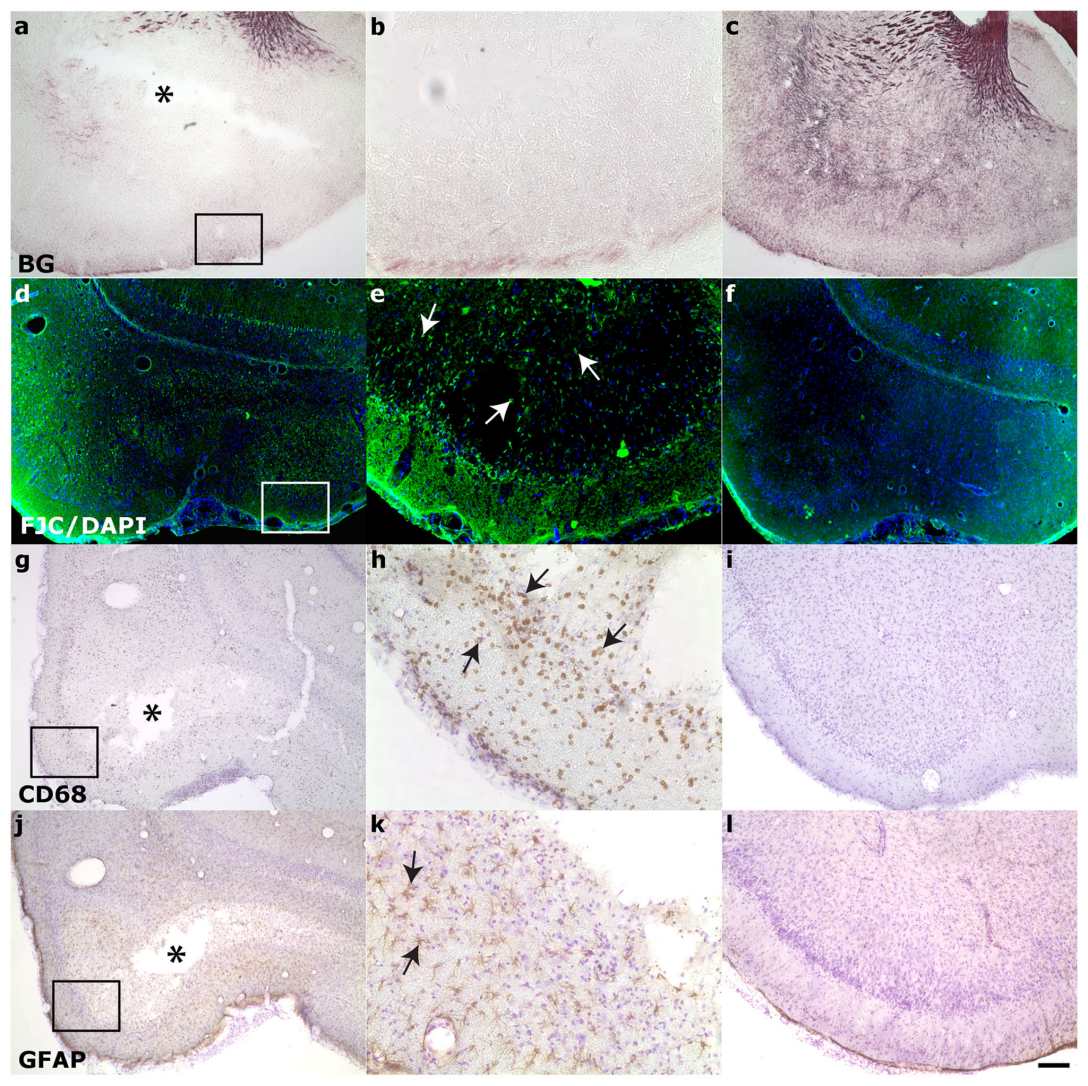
Characterization of parahippocampal damage 24 hours post status-epilepticus. Hyperintense regions observed in the parahippocampal region during in vivo T2-weighted imaging corresponded to A-C: myelin degradation, D-F: neuronal degeneration, G-I: macrophage activation, J-L: astrocytosis, and A,G,J: cavitation. Asterisks denote cavitation. Compare injured (left column:low magnification, middle column:high magnification) to uninjured (right column). BG = Black-gold II; FJC/DAPI = Flouro-jade C with 4',6-diamidino-2-phenylindole nuclear counterstain; GFAP = Glial fibrillary acidic protein; CD68 = Cluster of Differentiation 68; CV = Cresyl violet. Scale bar is 50µm for B,E,H,K; 200µm for all other images.
